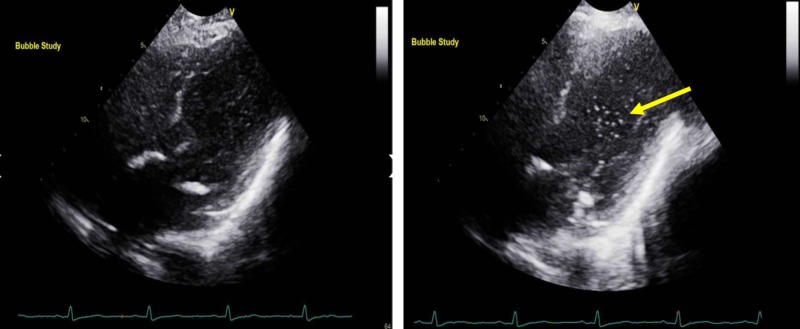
Transthoracic echocardiogram. Late opacification with microbubbles in the left heart chambers after five heart cycles are seen.
